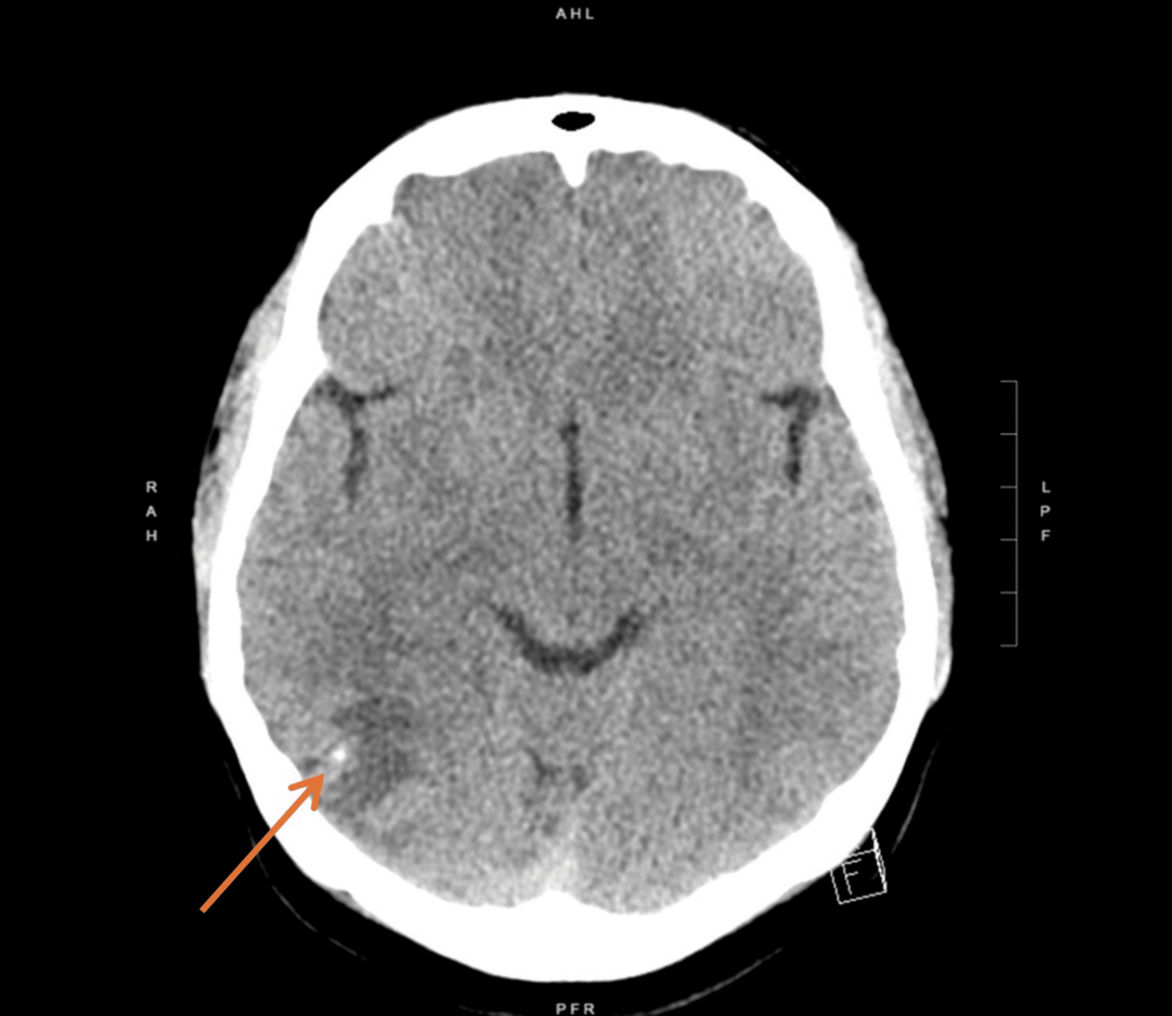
CT head without contrast scan showing a calcification in the right occipital lobe and vasogenic edema
